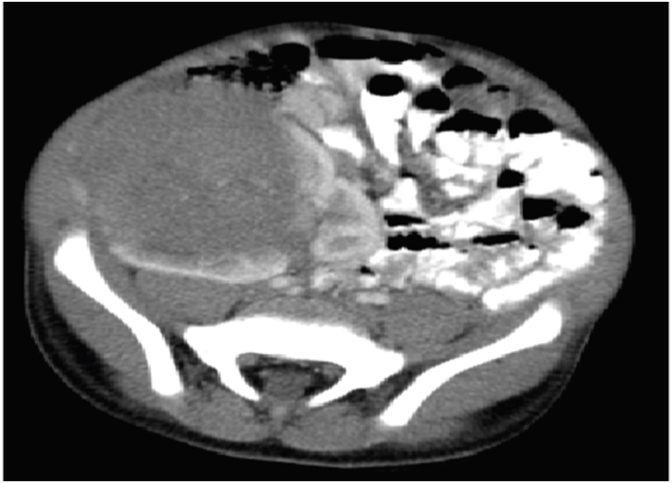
Computed tomography (CT) scan image of abdomen (coronal and transverse sections) showing tumors (red arrow) involving bilateral kidneys and compressed normal part of left kidney (black arrow). (For interpretation of the references to color in this figure legend, the reader is referred to the Web version of this article.)
